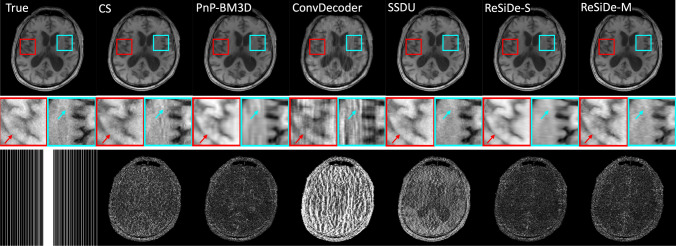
An example showing reconstruction of a T1-weighted image with sampling mask M1. To highlight differences, the second row magnifies two areas in the brain. The arrows point to features where some of the methods show artifacts or blurring. The third row shows the sampling mask (left) and the absolute error maps after five-fold amplification
